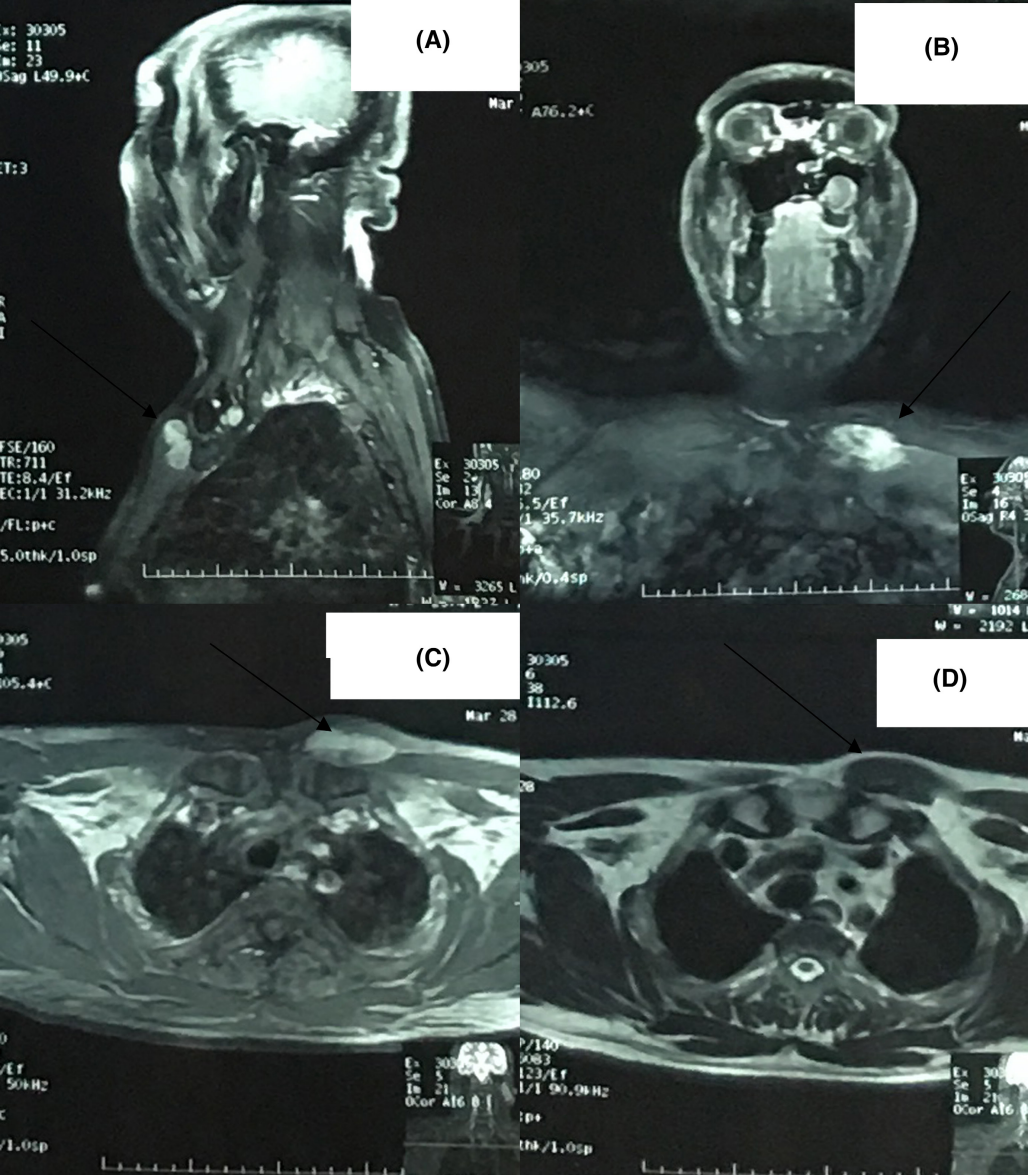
Magnetic resonance image of the chest and neck showing an abnormal signal intensity lesion identified subcutaneously at the level of medial end of clavicle causing a focal bulge anteriorly, abutting the left pectoralis major with no bony changes. (A) Sagittal sequence; (B) Coronal sequence showing neck; (C) Axial T1W imaging; (D) Axial T2W imaging.
